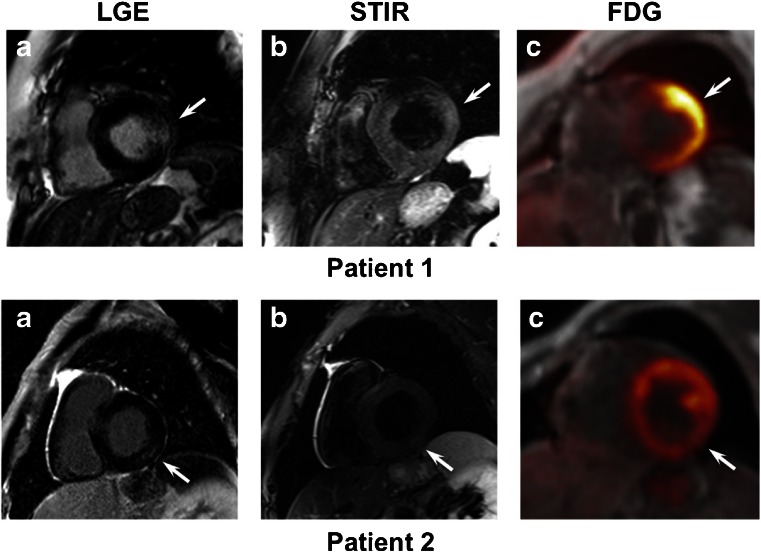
PET/MRI imaging in Anderson-Fabry disease [25]. *LGE* late gadolinium enhancement, *STIR* short inversion time inversion recovery
